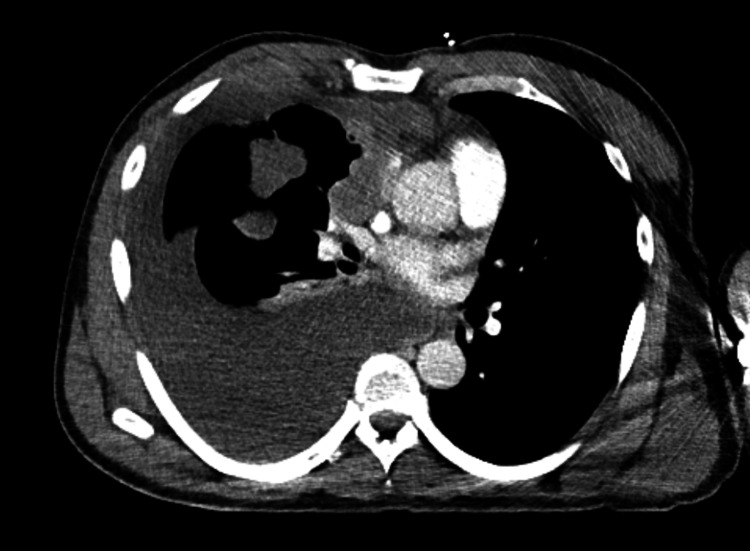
CT chest with contrast, axial view CT chest shows large confluent right hilar mass, mass-like pleural thickening in addition to nodular pleural thickening throughout the right hemithorax. Large right-sided pleural effusion with significant mass-effect and compressive atelectasis on the right lower and middle lung lobes.
